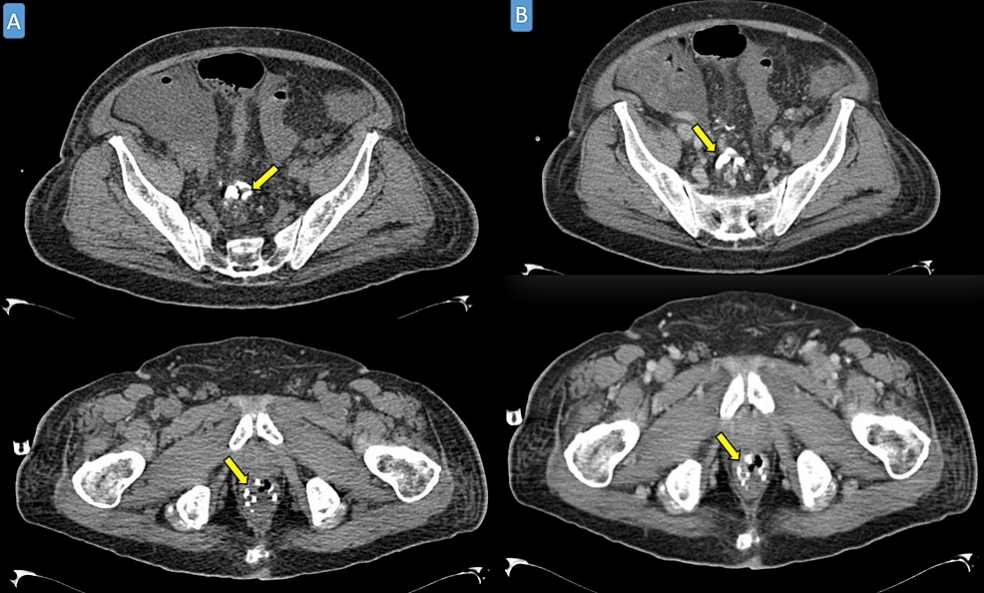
Post-procedure axial abdominal CT scan in plain (A) and post-contrast (B) displaying perirectal/perianal multiple hyperdensities of embolization materials (yellow arrows).
